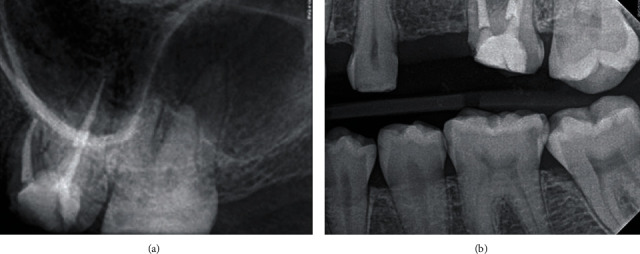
Postoperative intraoral radiographs. Intraoral periapical radiograph of the maxillary left first molar after root canal retreatment (a). Intraoral bitewing radiograph of the maxillary left first molar after root canal retreatment (b).
